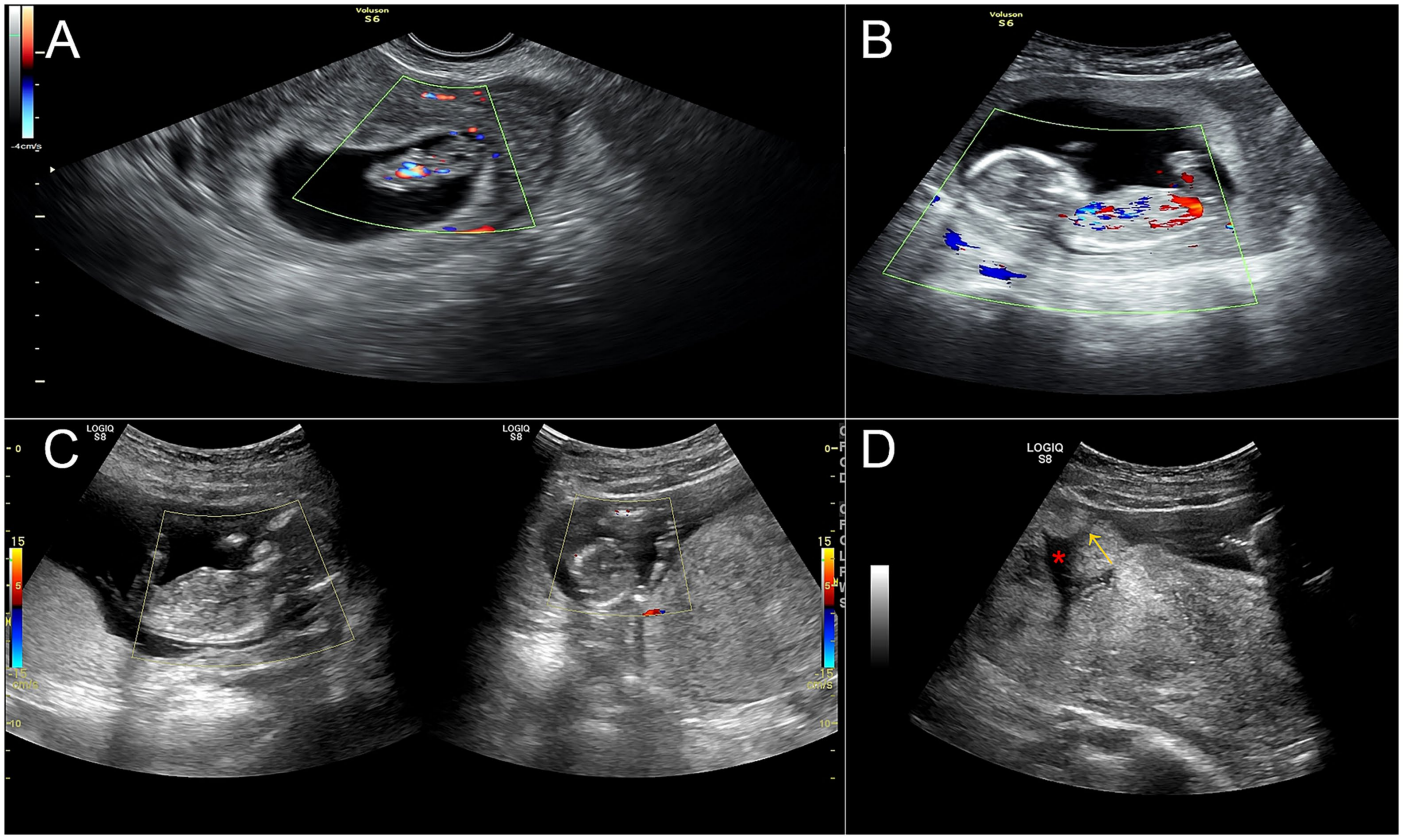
Ultrasound revealed the clinical course from ineffective evacuation to uterine rupture. (A) A continuing pregnancy after suction evacuation with a fetal pole measuring approximately 25 mm. (B) A 15-week fetus before uterine rupture. (C) A fetus without cardiac activity floating in the peritoneal cavity. (D) Myometrial discontinuity was noted at the uterine fundus, with a clear defect (red asterisk) and rupture site (yellow arrow).
